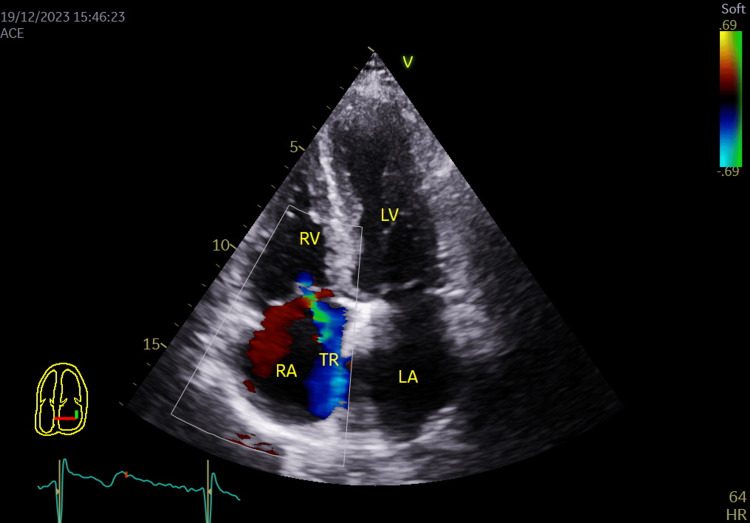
Color flow coding showing grade II tricuspid regurgitation in apical 4 chambers view. LA: Left atrium; LV: Left ventricle; RA: Right atrium; RV: Right ventricle; TR: Tricuspid regurgitation.
